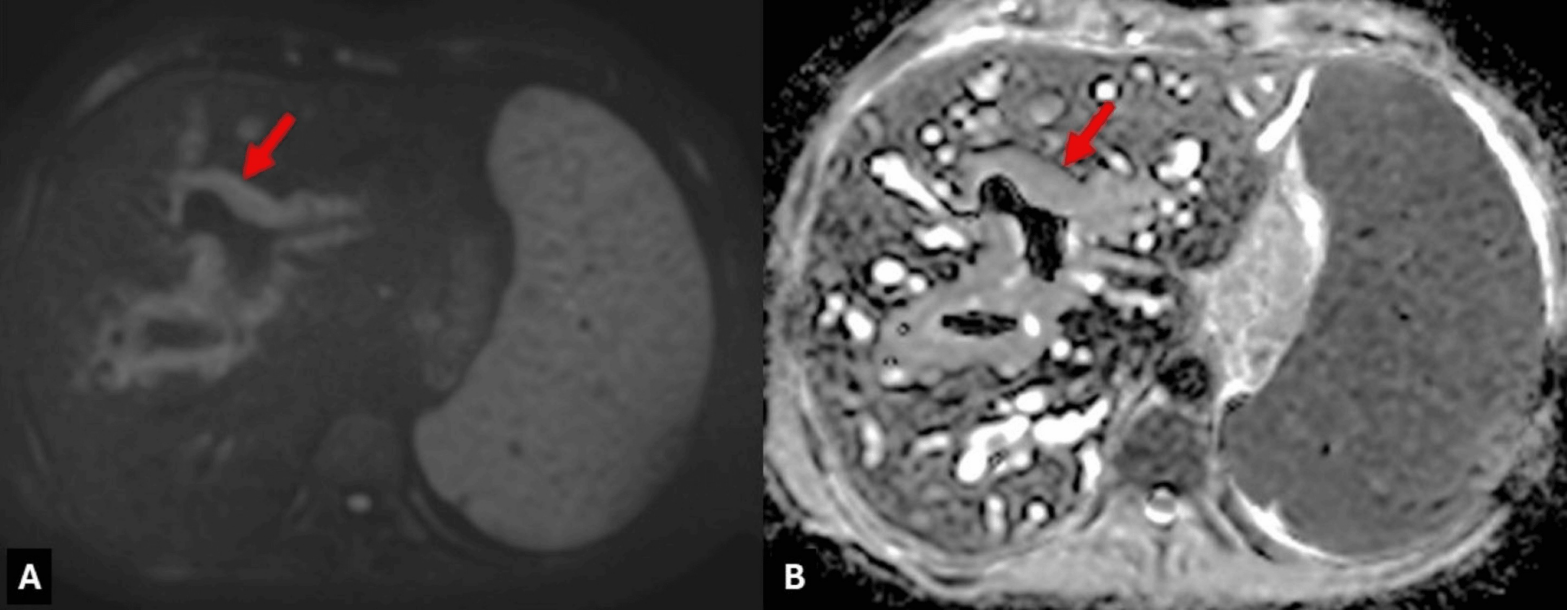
MRI diffusion sequences, B1000 and ADC map (A and B, respectively), show slightly hyperintense nodular periportal soft tissue (red arrows) without diffusion restriction. ADC: apparent diffusion coefficient
